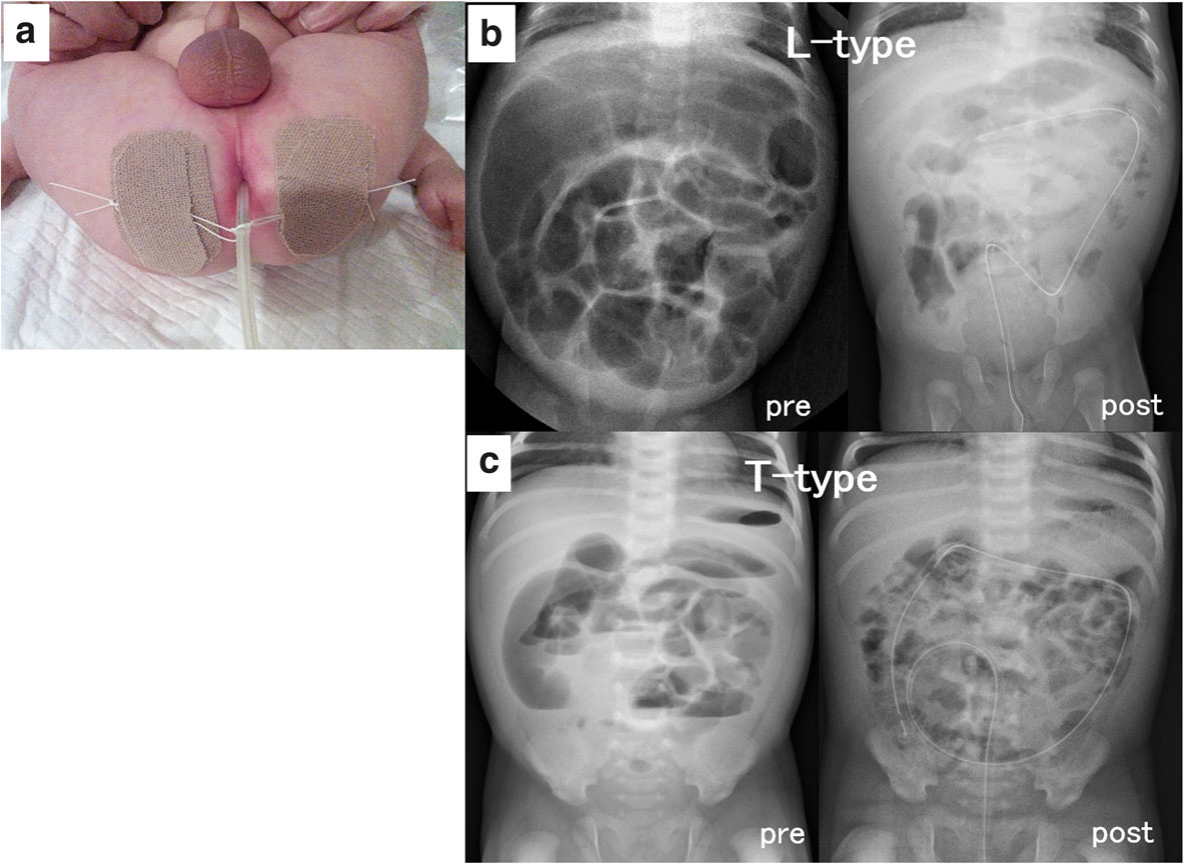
Indwelling transanal tube (ITT). a A 10–12F flexible drainage tube was placed and secured to the bilateral buttocks. b Decompression using an ITT in long-segment (L)-type Hirschsprung’s disease; abdominal X-ray taken before decompression showing a dilated colon (*pre*), which disappeared after ITT insertion (*post*). c Decompression using an ITT in total (T)-type HD; abdominal distention is visibly improved after (*post*) versus before (*pre*) ITT insertion
